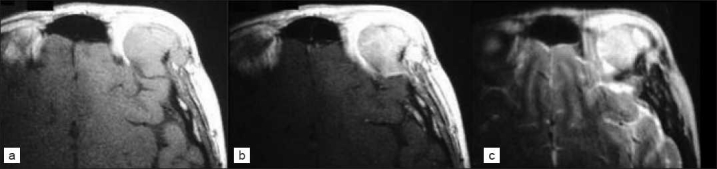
CT scan (a, b) postcontrast images showing enhanced intracranial mass of the supraorbital area with bone involvement and (c) bone window image showing bone destruction by the mass
